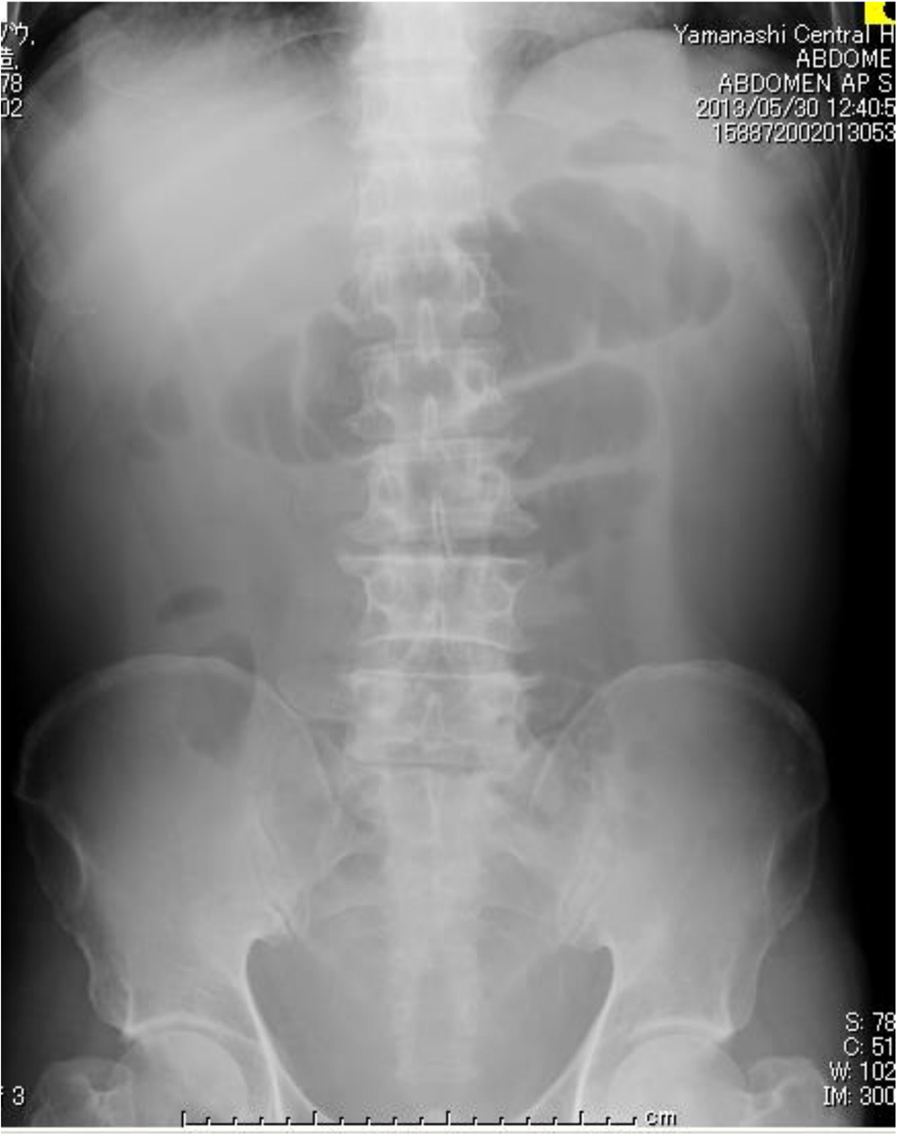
Abdominal X-ray on admission. The X-ray showed dilated small intestine indicating mechanical bowel obstruction
